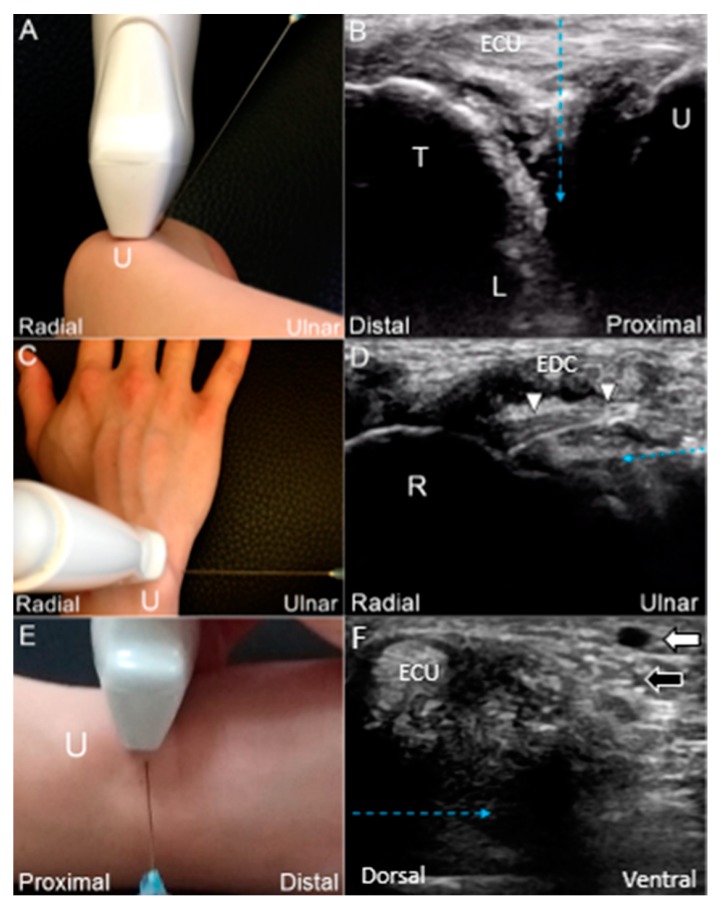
(A) US-guided injection using the *out-of-plane* approach. (B) The needle *(blue dashed arrow)* is advanced toward the radial aspect, deeper to the ECU tendon. (C) US-guided injection using the *in-plane* approach from the ulnar wrist. (D) The needle is advanced toward the radial aspect, deeper to the dorsal radioulnar ligament *(white arrowhead)*. (E) US-guided injection using the *in-plane* approach from the dorsal wrist. (F) The needle is advanced toward the ventral aspect, deeper to the ECU tendon. US: ultrasound; U: ulna; R: radius; L: lunate; T: triquetrum; white arrow: artery; black arrow: dorsal ulnar cutaneous nerve; ECU: extensor carpi ulnaris; EDC: extensor digitorum communis.
